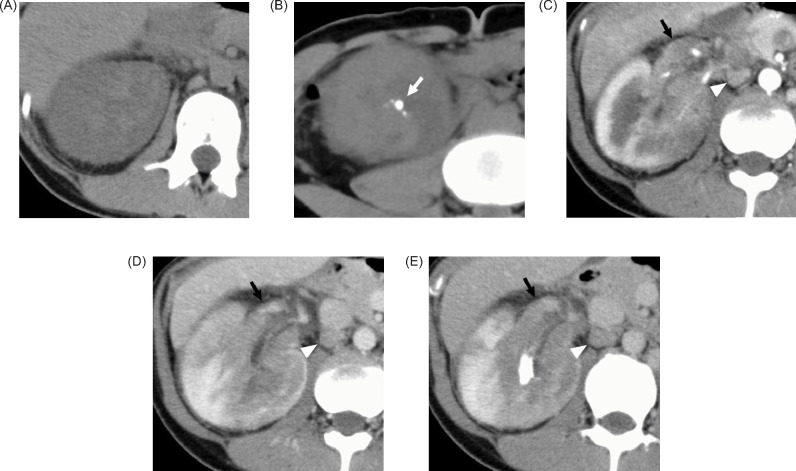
CT revealed the mass in the right kidney with a right renal vein thrombosis (black arrows). At non-enhanced CT (A, B), the mass was slightly hyperattenuating compared with the adjacent cortex with calcifications on the caudal side of the mass (white arrow). At dynamic contrast-enhanced CT (C, D, E), the solid portions of the mass were enhanced less intensely compared with the adjacent renal cortex and enhanced more intensely compared with the adjacent renal medulla during the corticomedullary (C) and nephrographic phase (D) of enhancement. The mass showed slight washout during excretory phase (E). There was lymphadenopathy approximately 14 × 8 mm in the right renal hilus (white arrowheads), and metastasis was suspected.
